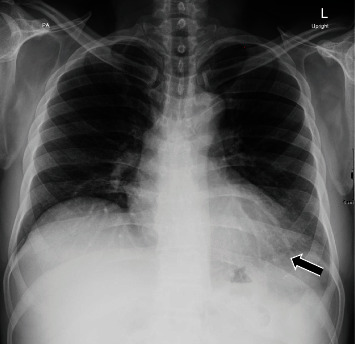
Upright PA chest X-ray showing faint opacity in the lower zone of the left lung.
